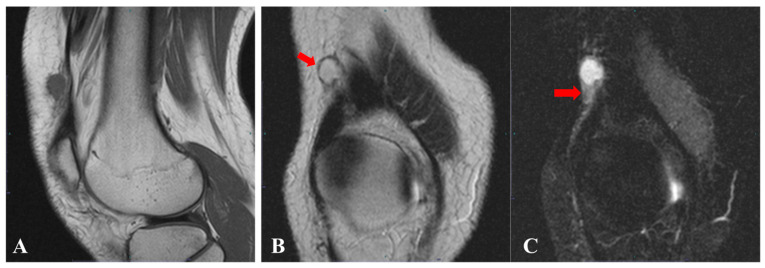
A 24-year-old female patient was referred to our hospital with a tumor in the proximal lateral aspect of the right knee. Eight years earlier, she had palpated a mass in the same location and visited a local clinic, where magnetic resonance imaging (MRI) was performed. MRI showed a tumor with iso-intensity signals on T1-weighted imaging (T1WI) compared with muscle, and high-intensity signals on T2-weighted imaging (T2WI) and fat-suppressed T2WI, measuring 12.5 × 10.5 × 9.5 mm (A). In addition, a pseudocapsule (B; red arrow) and peritumoral edema (C; red arrow) were identified. At that time, contrast-enhanced MRI was not performed. Based on these findings, a benign cystic tumor, such as ganglion or bursitis, was suspected, and careful follow-up was recommended.
